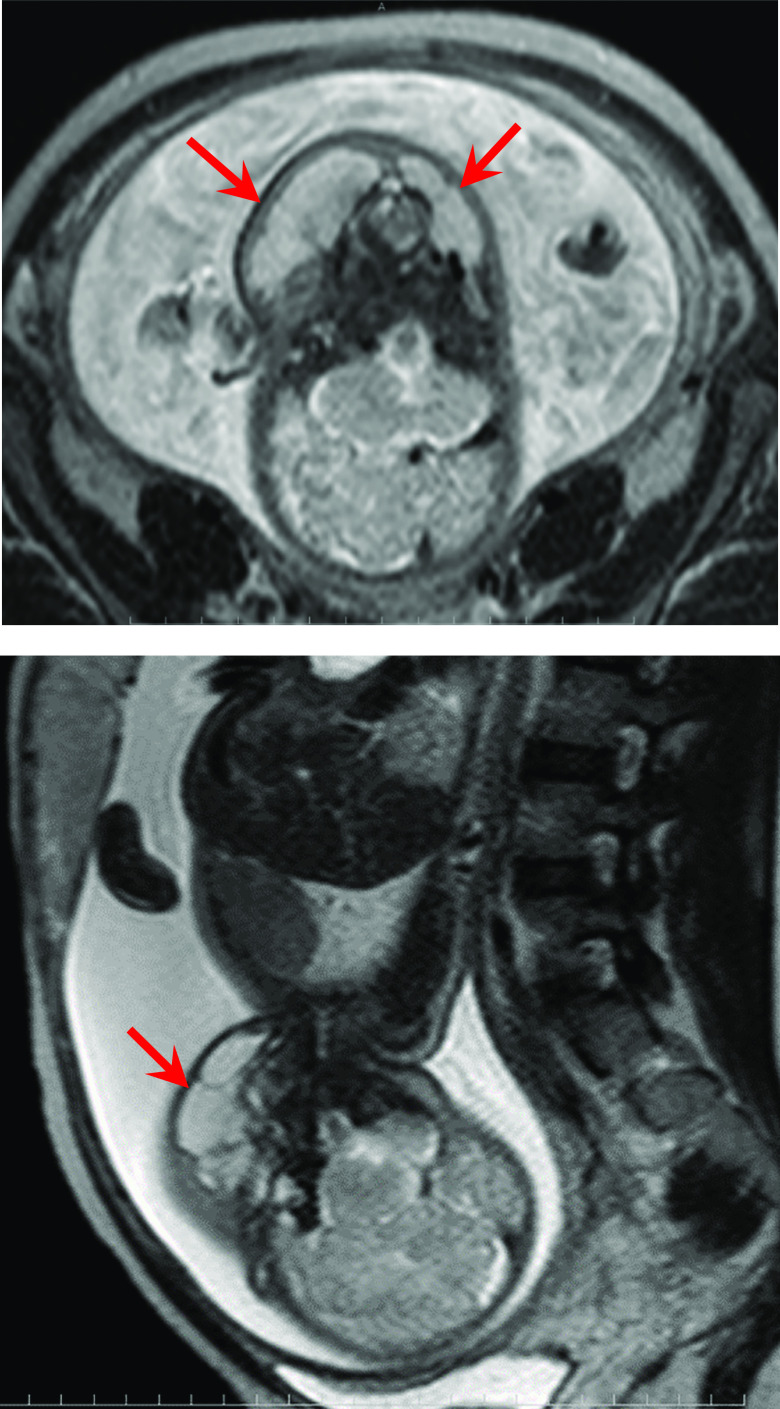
T2-weighted MRI T12-weighted Image with high intensity on the second day of the 35th week of pregnancy (A: T2 Axial, B: T2 Sagital) Multilocular cystic mass lesions having almost internal uniformity were observed in the bilateral cervixes
